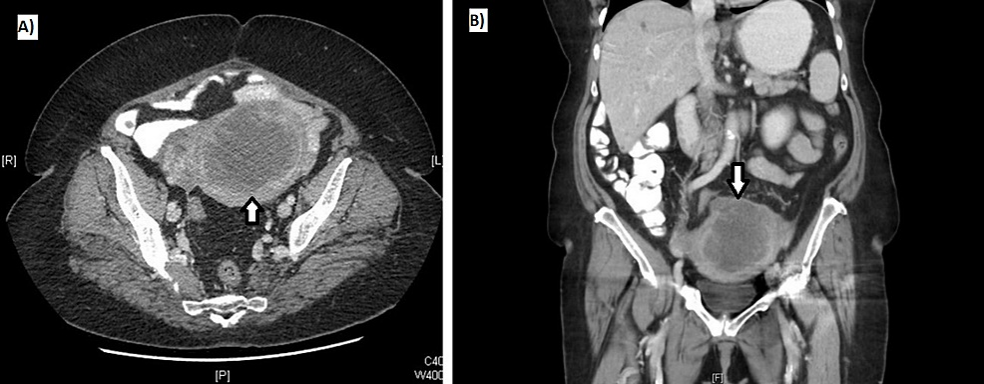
Abdominopelvic CT scan after administration of oral Gastografin and intravenous iodinated contrast in the venous phase A) and B) Anteverted uterus with great distention and occupation of the endometrial cavity by a mass with a neoplastic appearance that infiltrates more than 50% of the thickness of the myometrium, as well as the uterine serosa, with an exophytic growth pattern, which contacts a sigmoid segment, without fat cleavage plane and with small inflammatory changes in the adjacent fat, not being able to rule out tumor infiltration of the fat.
